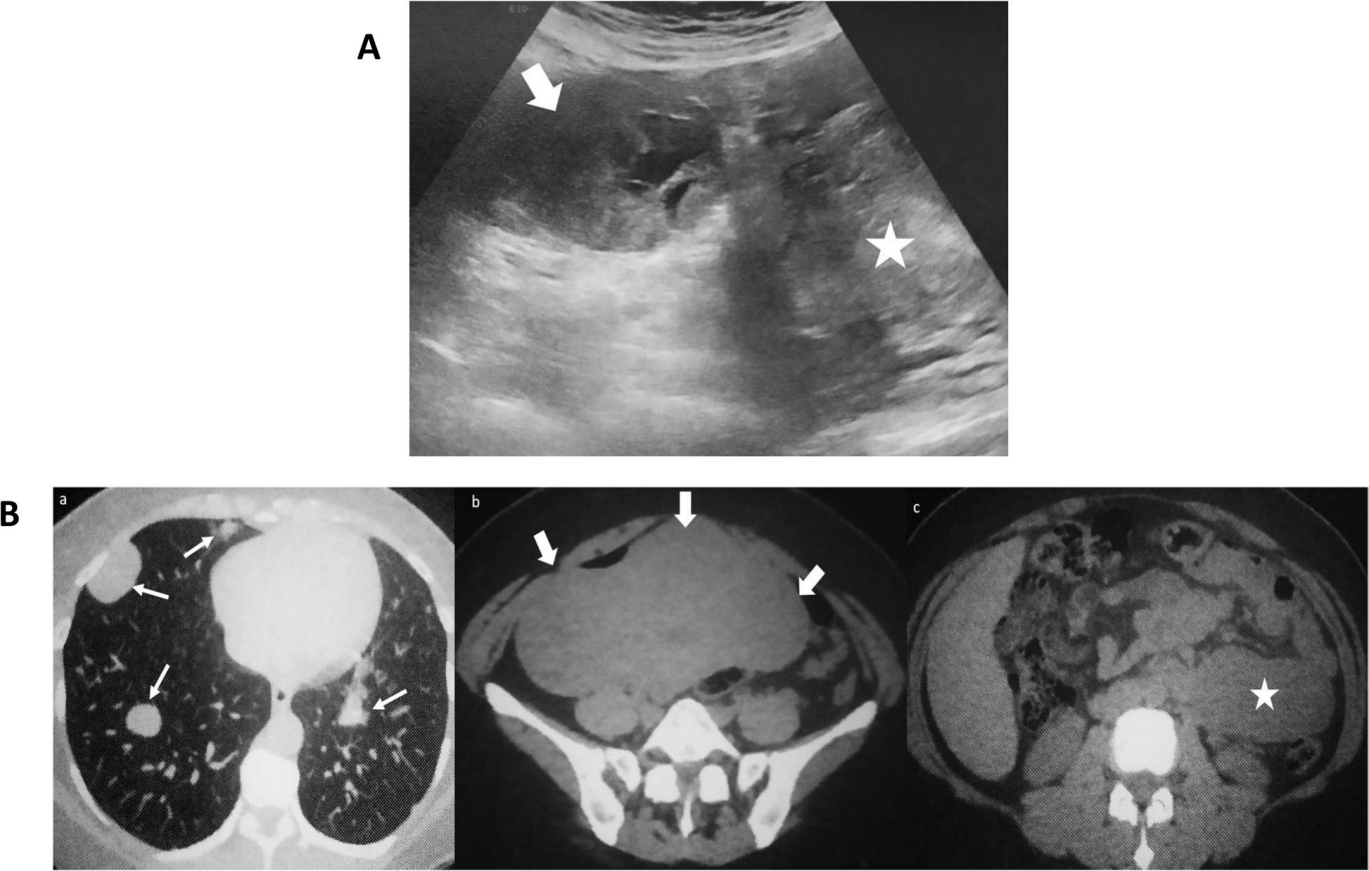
A An ultrasound image of a heterogeneous hypoechoic subserosal nodule (arrow) in the uterus (indicated by ★) is shown. B A non-enhanced CT scan demonstrating (a) many well-defined lung nodules (arrows), (b) multiple uterine fibroids (thick arrows), and (c) a mass in the left hypochondrium (indicated by ★)
